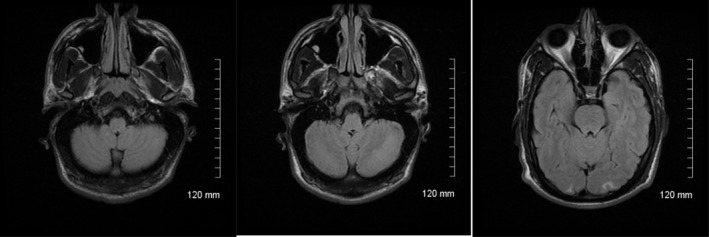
MRI FLAIR sequence on day 5
